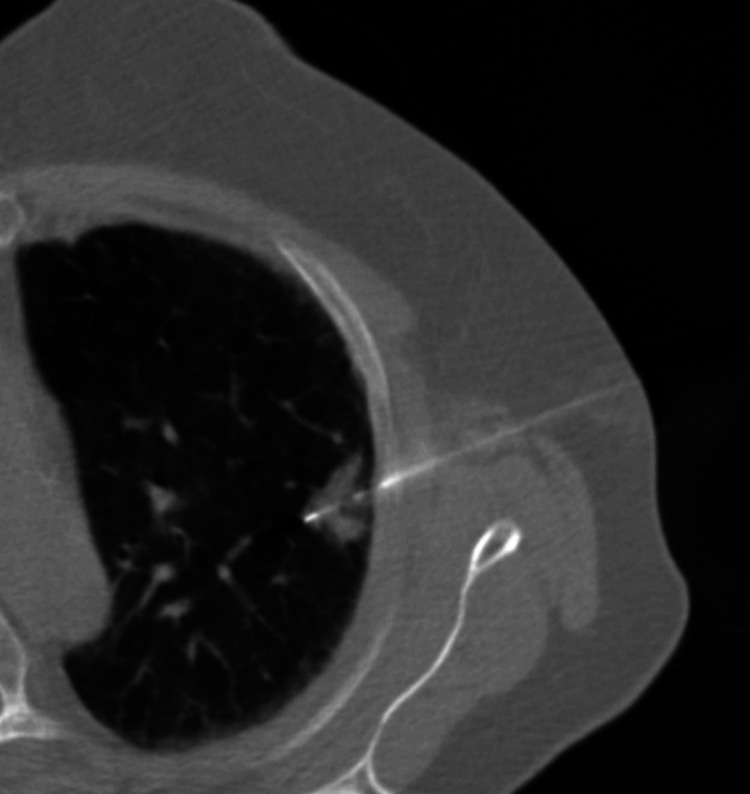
CT-guided biopsy of left upper lobe nodule CT: computed tomography
